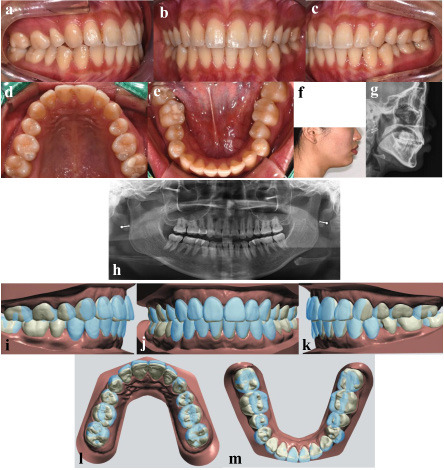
Diagnosis and virtual treatment plan. Pretreatment intraoral and facial photos, and cephalometric and panoramic radiographs (a-h). Treatment setup (i-m); Pretreatment occlusion is shown in blue, and the planned occlusion is shown in white.
